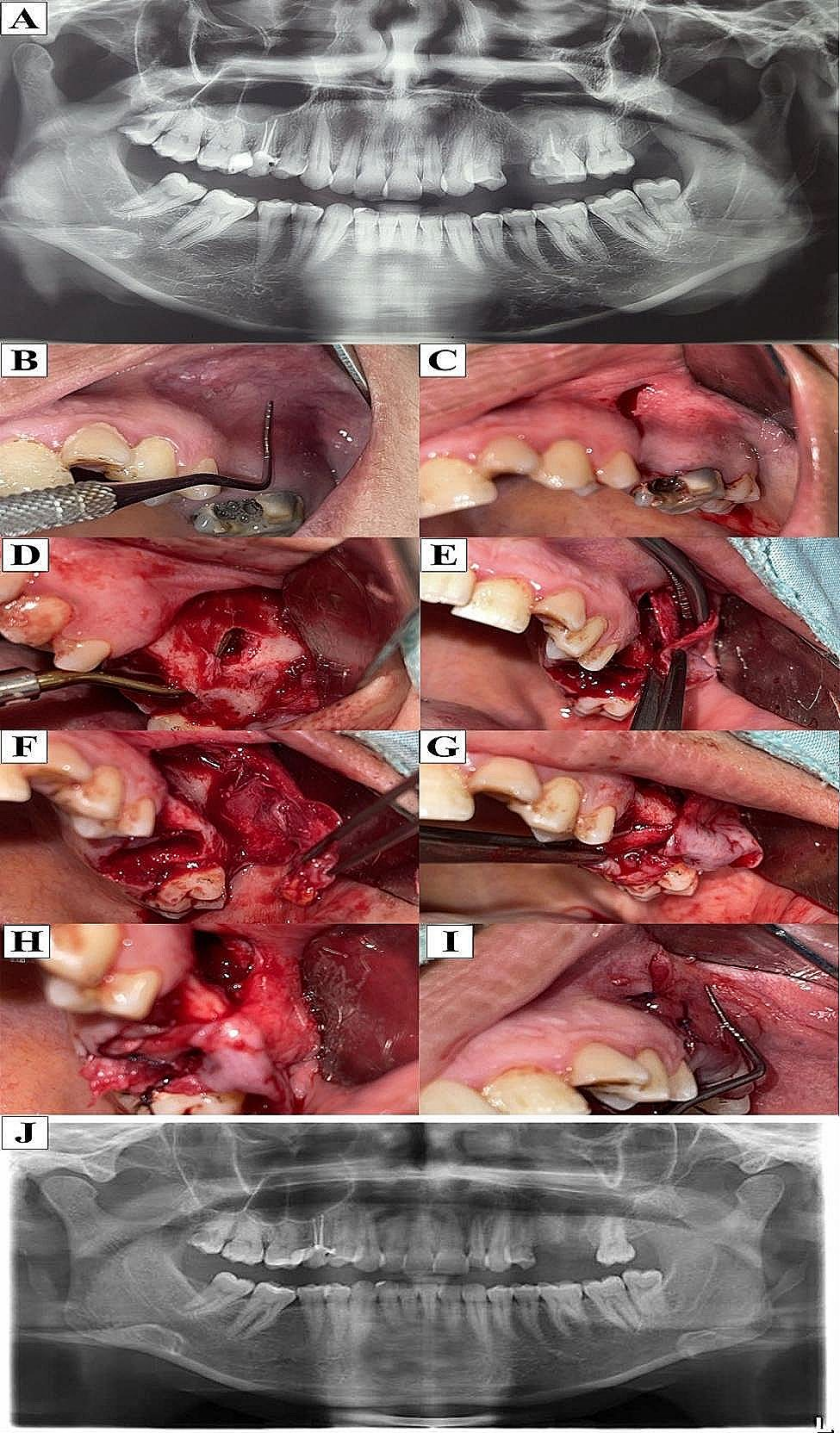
(A) Preoperative panoramic X-ray film (B) Preoperative photograph showing the vestibular depth of buccal sulcus before extraction of upper left first molar (C) Incision of buccal mucosal flap, (D) Reflection of the flap showing bony defect related to the oroantral fistula of upper first molar, (E, F,G) Dissection of periosteal flap from the overlying buccal mucosa, (H) Suturing of the periosteal flap over the oroantral fistula, (I) Suturing of the buccal mucosa to the palatal tissue with preservation of vestibular depth (J) Postoperative panoramic X ray, Case No 9
